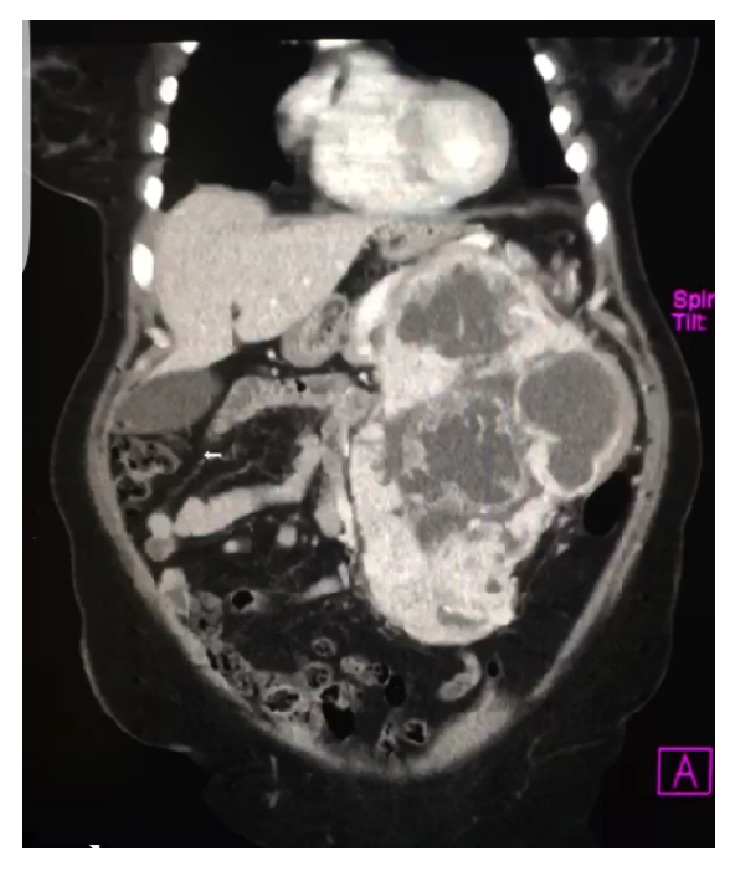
CT scan showing a 23 cm, thick-walled, multicystic mass occupying most of the left upper quadrant of the abdomen.
